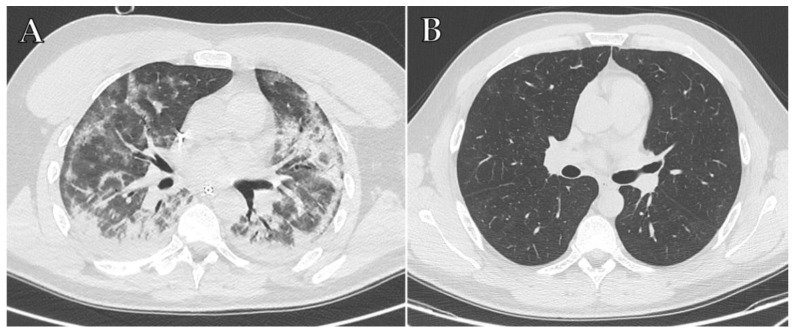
Axial HRCT images of a 38-year-old man with COVID-19 ARDS admitted to ICU at the same level, performed at different times: baseline scan (A) and 7-month follow-up (B). The baseline scan (A) shows typical imaging features indicative of severe COVID-19 pneumonia, including extensive bilateral parenchymal consolidations, mainly affecting the posterior regions of lower lobes, bilateral focal ground-glass opacities in the anterior regions and patchy consolidation, peripherally distributed, resembling pulmonary fibrosis. The 7-month scan (B) shows a complete resolution of the parenchymal consolidations and the apparent fibrotic abnormalities.
